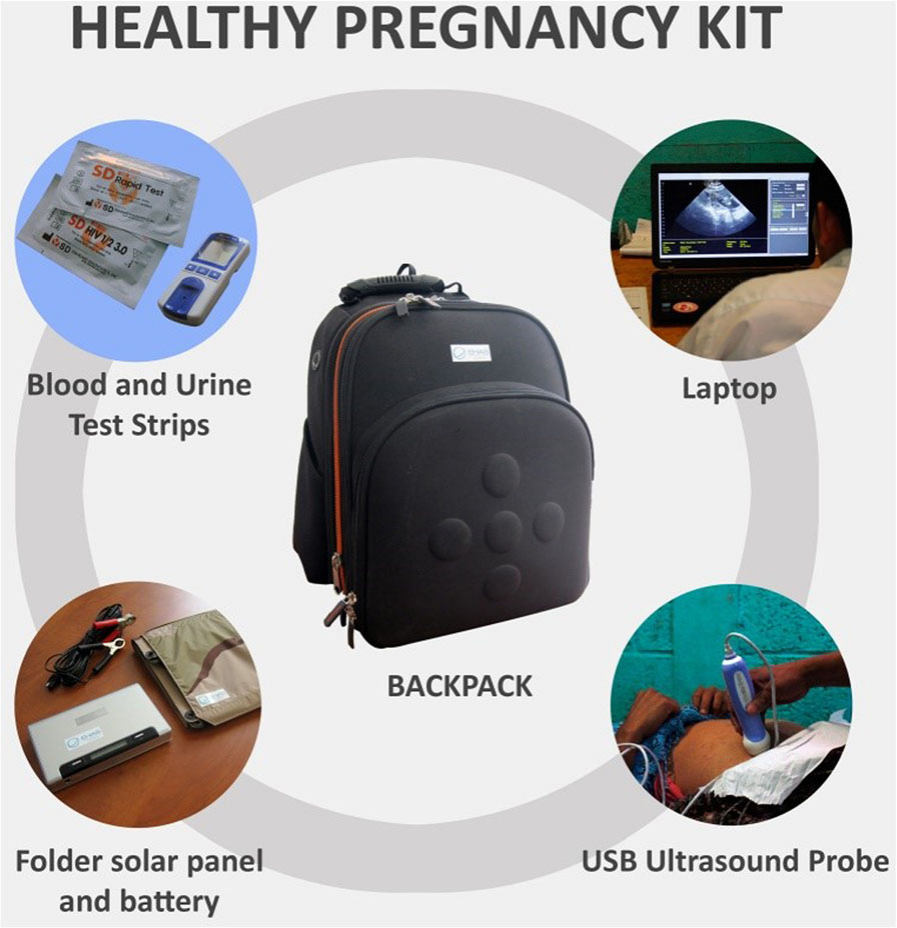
Materials of the prenatal control kit *Healthy Pregnancy.* A backpack containing a laptop, USB ultrasound probe, blood and urine rapid tests, two external batteries and a folding solar panel
